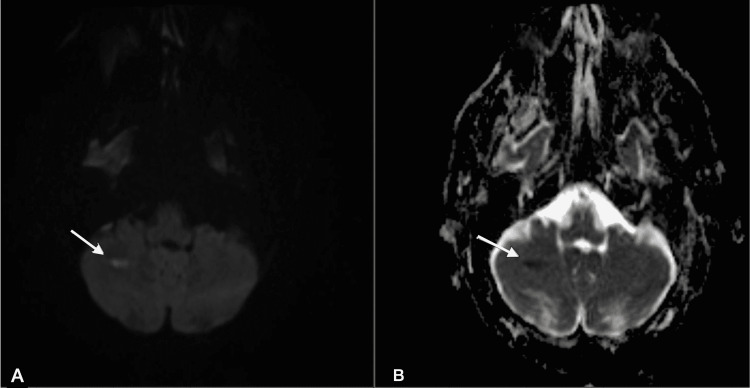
Diffusion-weighted MRI (A) and ADC map (B) shows focus of diffusion restriction with corresponding hypointensity on ADC map (white arrows), suggestive of acute lacunar infarct in the right cerebellar hemisphere.
